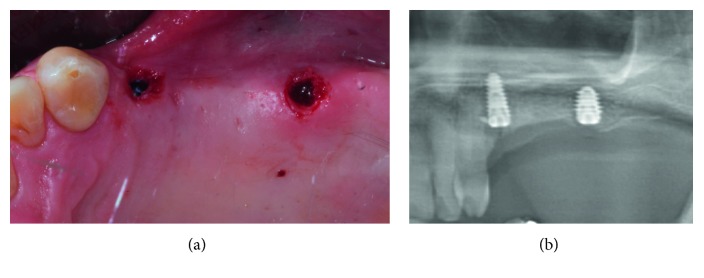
(a) Dental implant placement at the area that was expanded and augmented. (b) Section from panoramic radiograph representing the target site after dental implant placement.
